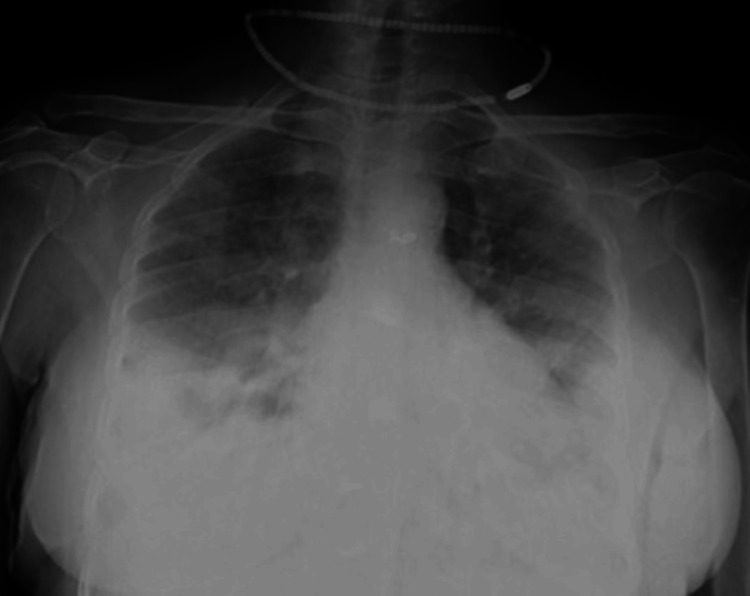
The supine chest radiograph (anteroposterior (AP) view) of a 64-year-old female patient revealed multiple confluent fluffy radiopacities in the bilateral lower lung zones, with peripheral predominance. Ill-defined ground glass opacities were seen in the medial parts of bilateral lower lung zones and bilateral mid-zones. Radiographic disease score=4 (moderate grade) as involvement was noted in both sides was 25% to 50%.
